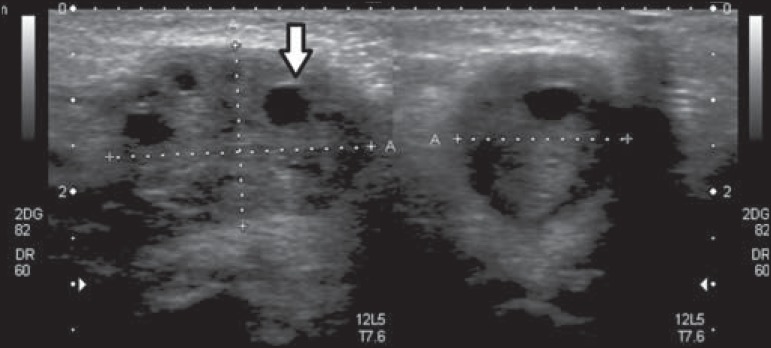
Ultrasound of the right labia majora. Note the right ovary, which is
